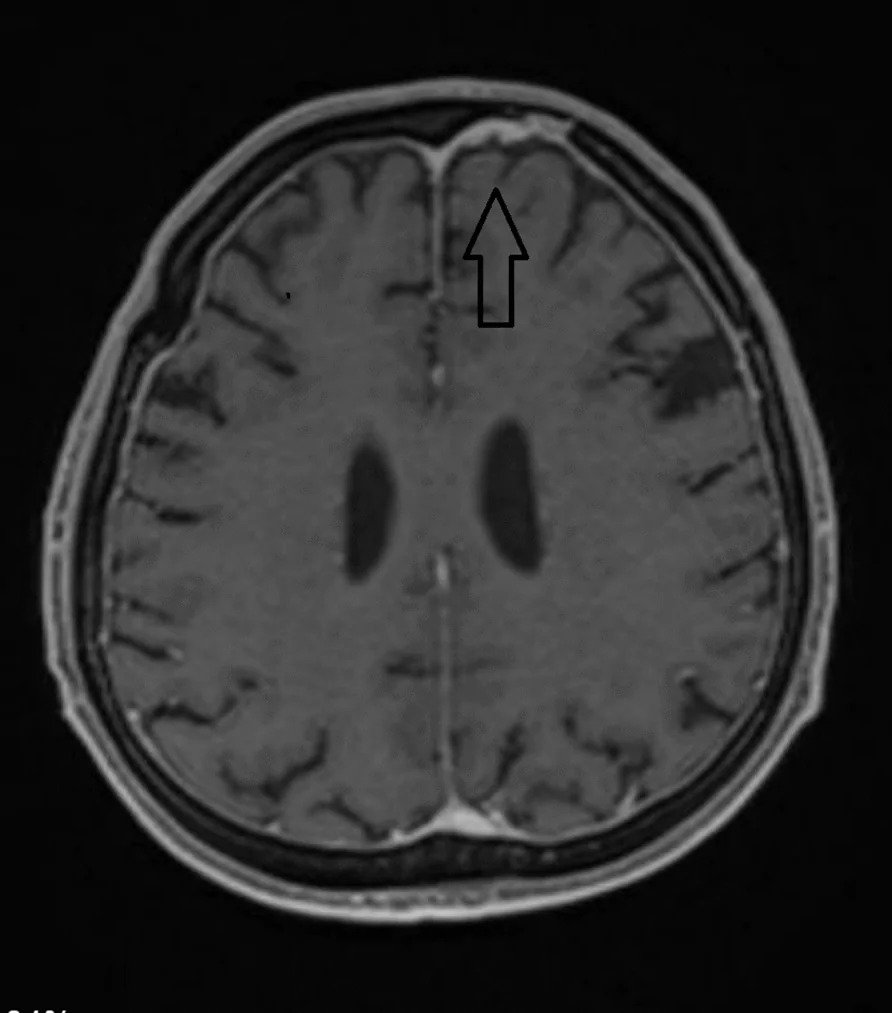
Brain MRI of Patient 2 showing thickening and enhancement of the dura mater with contrast medium enhancement (arrow)
